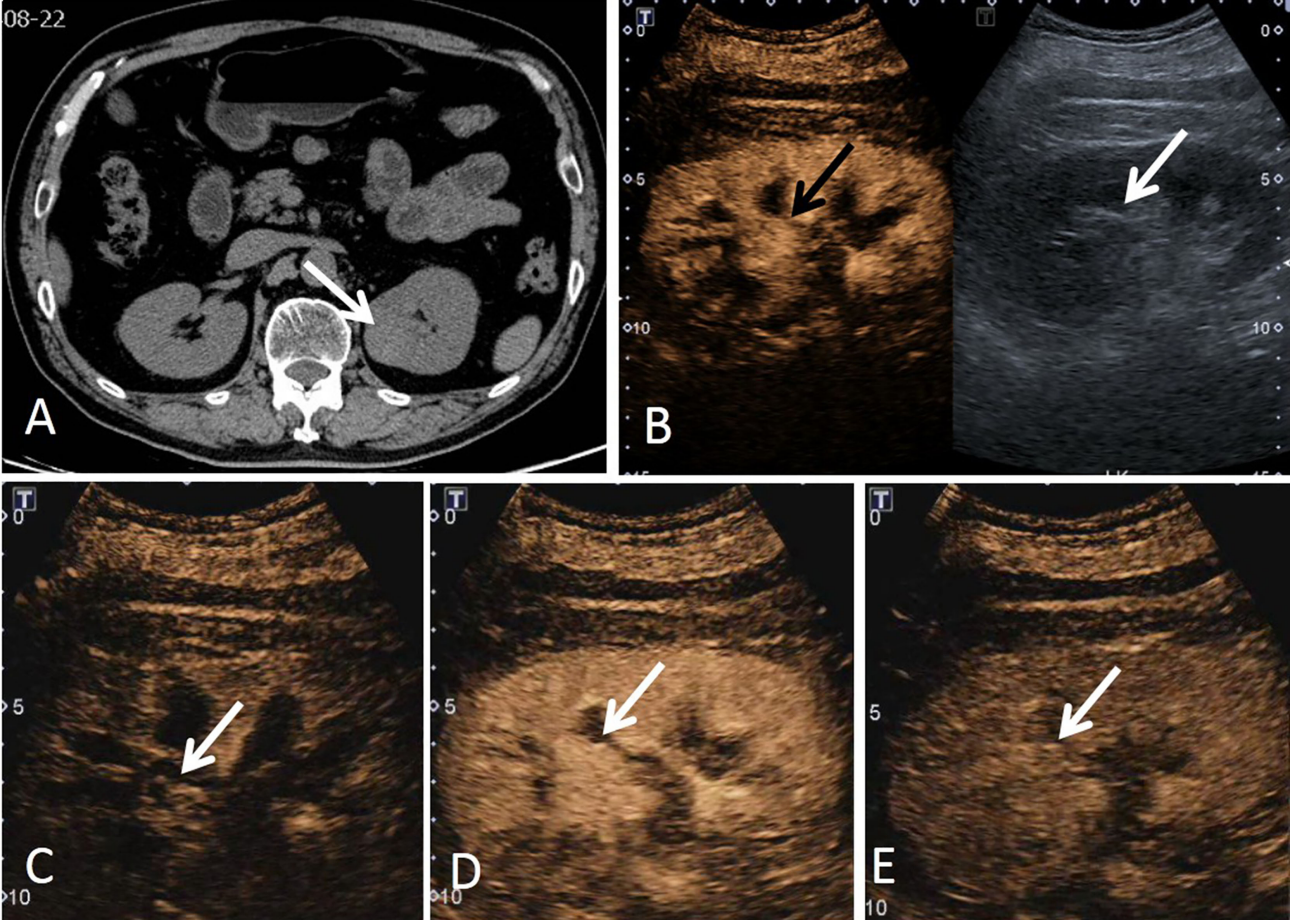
A 69-year-old man had a ccRCC in the left kidney with the size of 2.9 × 2.1 cm. (A) Non-enhanced CT scan showed a mass (white arrow) that was poorly demarcated from the renal pelvis and surrounding vessels. (B) According to the position displayed on CT, the simultaneous display mode of CUS (right) and CEUS (left) was performed, CEUS showed a suspicious mass with hyperenhancement (black arrow), but it was not clear on CUS (white arrow). (C) On CEUS imaging, the contrast agent entered the mass (white arrow) synchronously with the adjacent renal cortex. (D) The tumor showed homogeneous enhancement, and the enhancement intensity was slightly higher than that of the adjacent renal cortex (white arrow). (E) CEUS imaging showed that the tumor had a slow-out pattern (white arrow) compared to the renal cortex.
